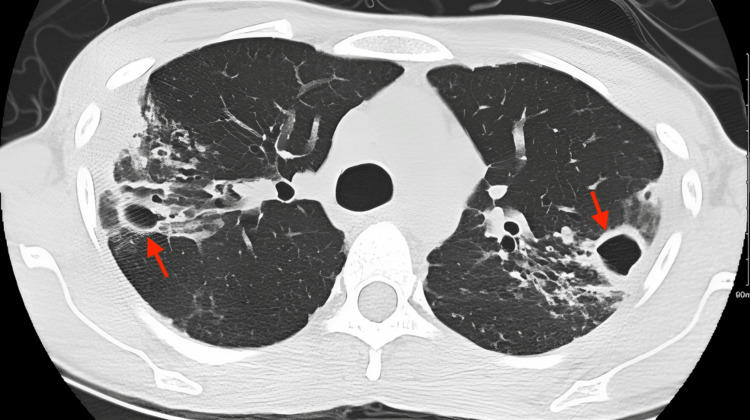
Chest CT showed bilateral nodular bronchiectasis predominantly in the bilateral upper lobes associated with cavitary lesions in the bilateral upper, bilateral lower, and right middle lobes, with the largest in the right upper lobe measuring 2.4 x 1.8 cm. CT: computerized tomography
